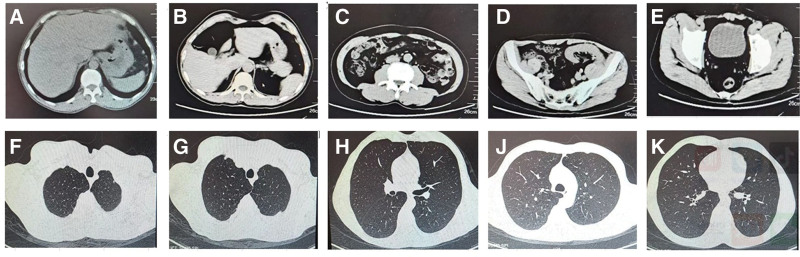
Changes of chest and abdomen CT at admission. (A–E) Changes of Abdominal CT at admission. (F–I) Changes of chest CT at admission.
